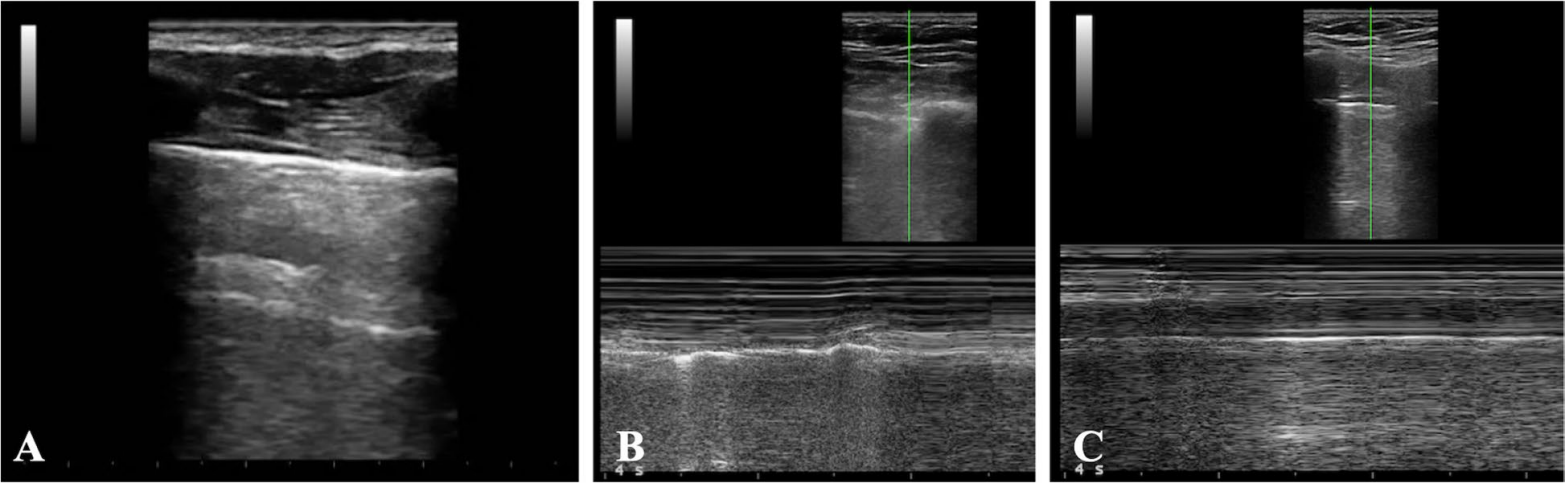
False lung sliding during Muller and Valsalva maneuvers. A Placing the probe perpendicular to two consecutive ribs in the parasternal area allowing visualization of the so-called “bat sign”: the upper and lower ribs are the wings of the bat, and, a little deeper, the pleural line is the body of the bat. B
